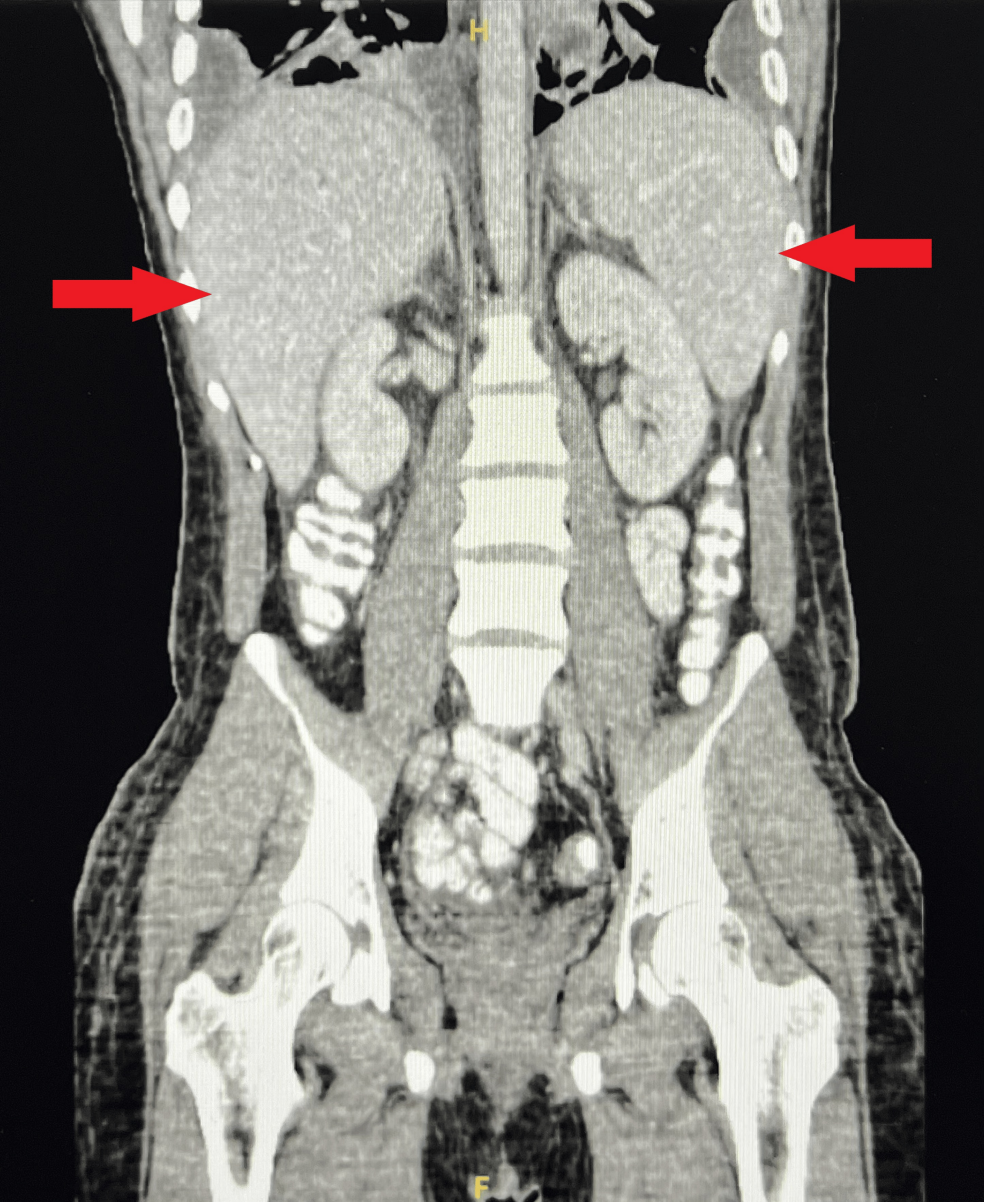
Computed tomography (CT) of the abdomen and pelvis showing hepatosplenomegaly, indicated by red arrows.
